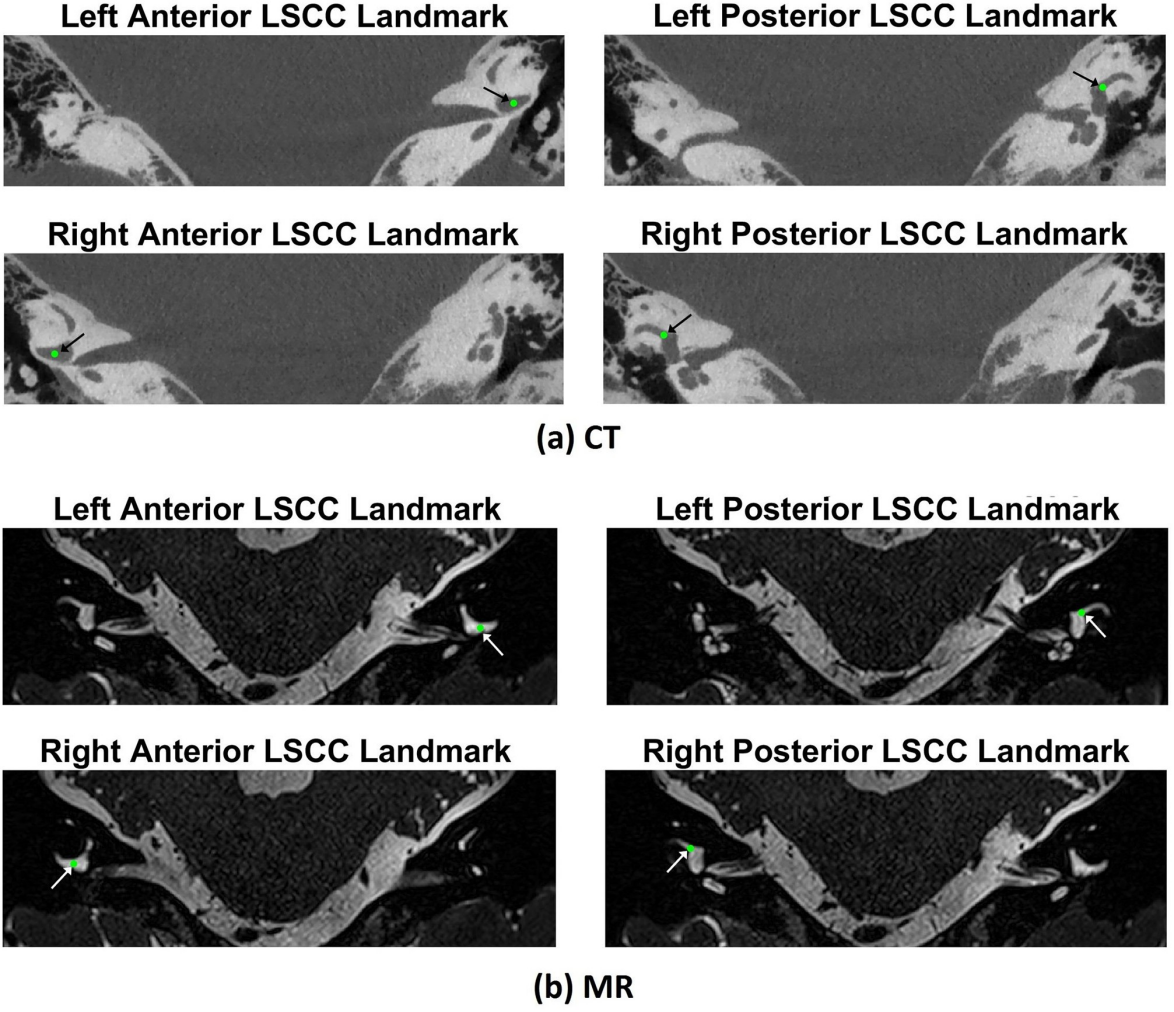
Lateral semi-circular canal (LSSC) landmarks selected from axial slices of the image stacks: (a) CT and (b) MR of the same patient. The landmarks are shown as green dots. The images have been cropped around the region of interest for clarity. The coordinates were obtained from a right handed system where the axes take the following directions. X: right to left, Y: anterior to posterior, and Z: inferior to superior. The four images of each image modality correspond to the axial slices at the Z coordinates of the selected points.
